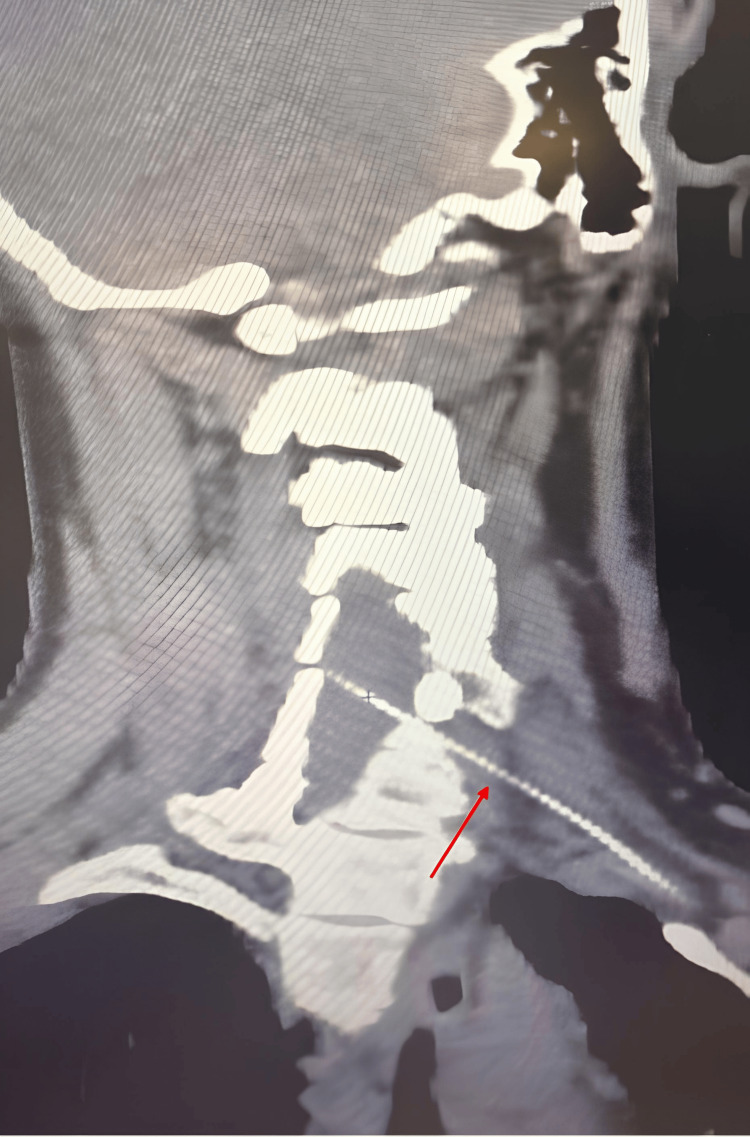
CT scan performed four years after thyroidectomy, showing the foreign body (red arrow) extending from the left subclavian artery toward the spinal cord
